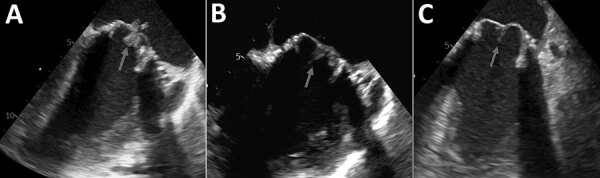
Transesophageal echocardiograph findings from a case of human *Lactococcus garvieae* bloodstream infection complicated by spondylodiscitis, Germany. A) Echocardiograph obtained on day 6 demonstrates mobile vegetations on both leaflets of the biological prosthetic mitral valve with a maximum dimension of 6 × 16 mm and pronounced valvular leaflet thickening (arrow). No notable valvular dysfunction was present. B) Echocardiograph obtained on day 45 shows marked regression of mitral valve leaflet thickening and a smaller vegetation (arrow; maximum 4 × 4 mm). No notable valvular dysfunction was present. C) Echocardiograph obtained on day 98 shows marked regression of mitral valve leaflet thickening. Arrow shows a faint 5 mm filamentous structure that might represent a residual finding. No notable valvular dysfunction was present.
